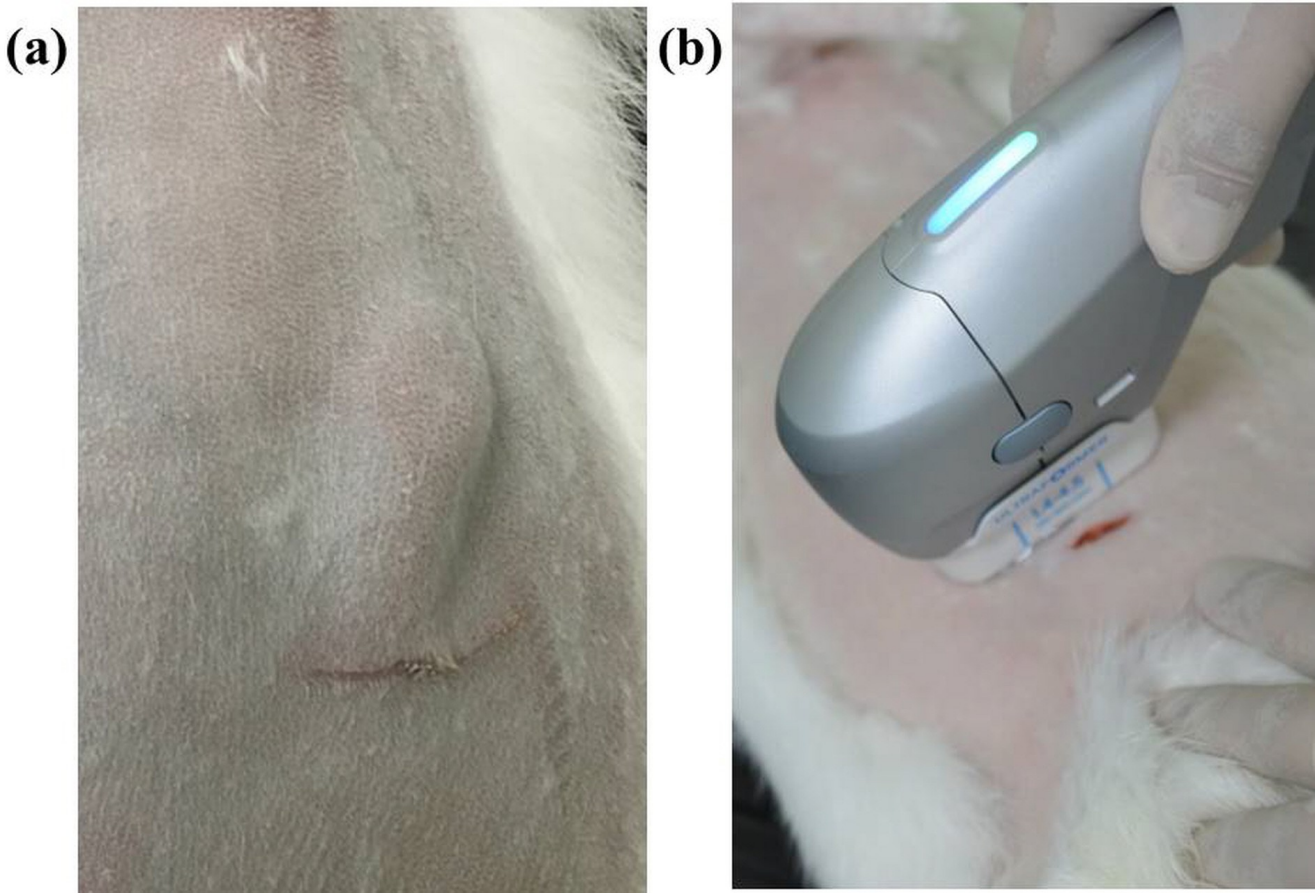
High-intensity focused ultrasound (HIFU) treatment after silicone injection. (a) After making a slit incision on the shaved skin of each rabbit, 1–2 cm^3^ of silicone was injected into the subcutaneous layer. (b) By using HIFU at a depth of 4.5 mm and a power of 1 J, the experimental group was subjected to mechanical and thermal damage at the silicone injection site.
